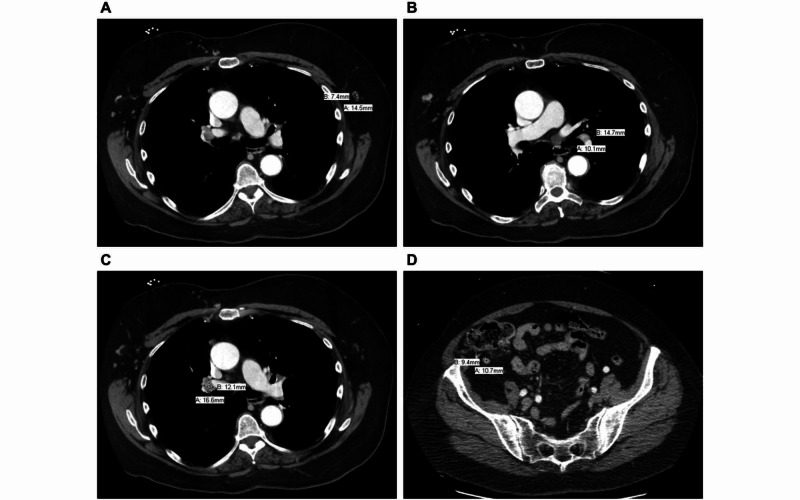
Various CT scan views display (A) axillary lymphadenopathy (axial view), (B) hilar lymphadenopathy (axial view), (C) mediastinal lymphadenopathy (axial view), and (D) mesenteric lymphadenopathy (axial view).
